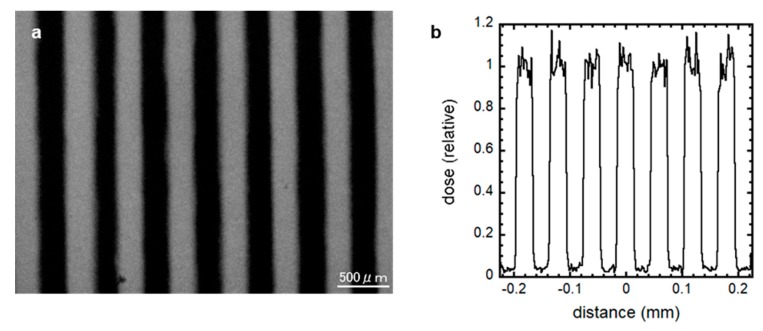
300 μm-slit-modulated beam dose profile. The modulated X-ray beams were created by shielding 50% of the cell culture using a 300 μm-slit Pb shielding material, and the dose distribution was confirmed using a Gafchromic XR-RV3 radiochromic film (a) and PHITS code ver. 2.96 (b). The irradiated regions in this optical image are shown as a black striped pattern. Scale bar, 500 μm.
